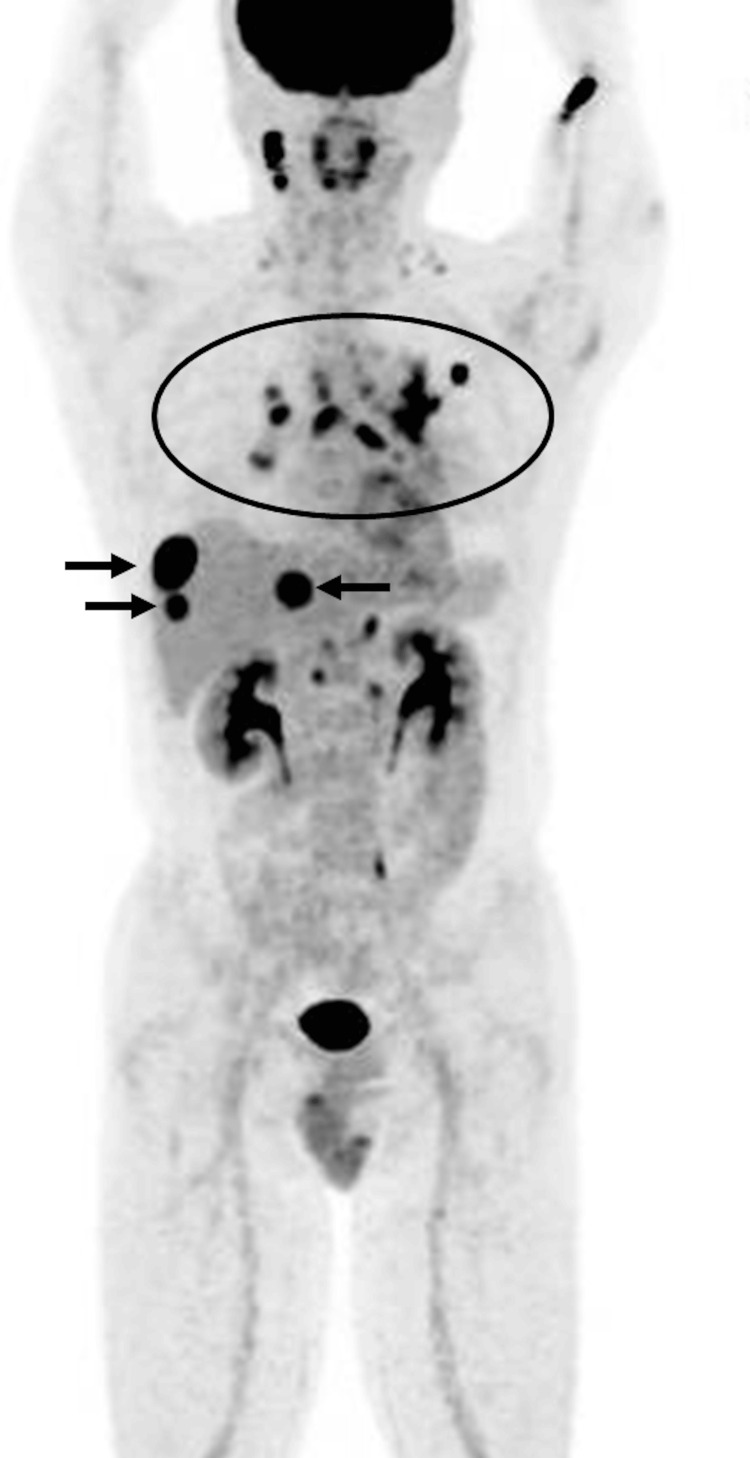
The FDG-PET showed metastases to mediastinal and hilar lymph nodes (circle), and liver (arrows). FDG-PET: 18F-fluorodeoxyglucose-positron emission tomography
